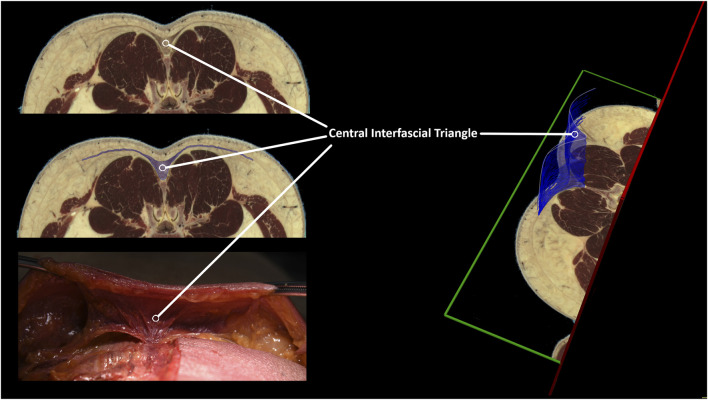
Identification of a central interfascial fascia triangle between the skin, erector spinae muscle, and lumbar spine. The lumbar tissues in the lumbar spine were examined using an MRI anatomical model (Visible Human Male) (NIH, 2013). Regions of interest were defined, encompassing the lumbar central interfascial triangle, which was manually segmented with transverse anatomical cross-references from the upper endplate of L2 to the lower endplate of L3. The 3D representation of the central interfascial triangle is the result of our segmentation, spanning from L3 to L2. Although not shown in this figure, this triangular-shaped tissue is present from S1 up to T12. The segmentation was performed using MITK v2022.10.
